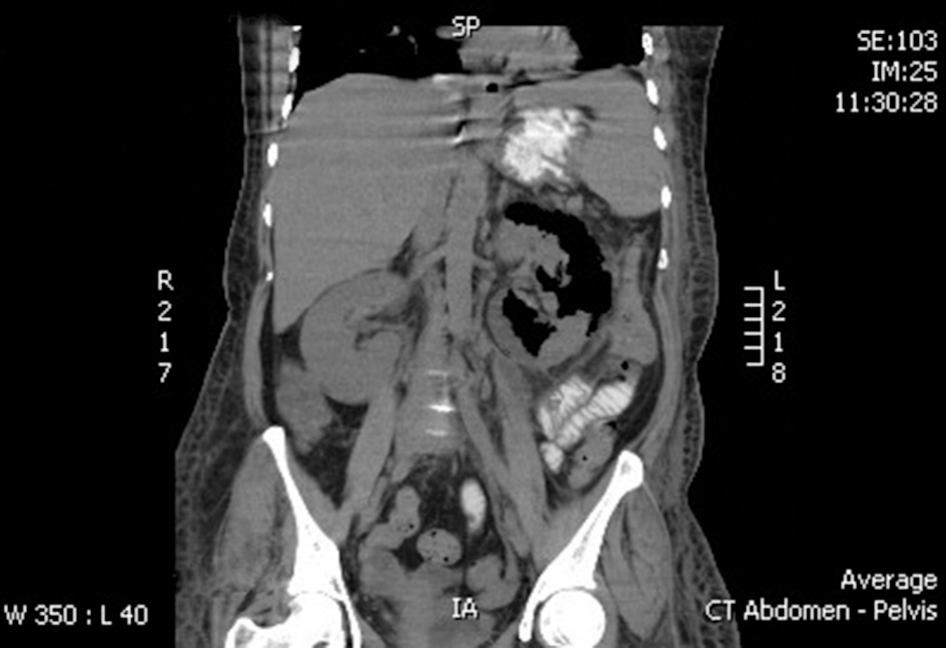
CT Features of Left Sided Emphysematous Pyelonephritis (Coronal Cut)
